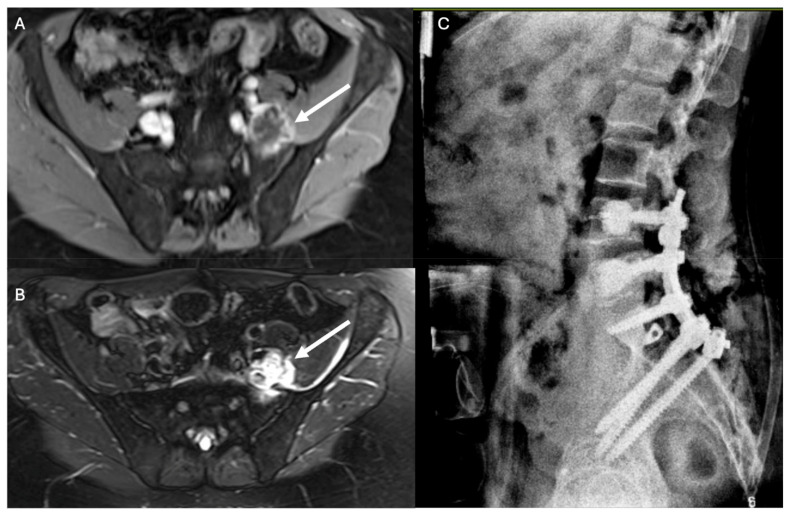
Case 3: pre- versus postoperative imaging. Preoperative T1 post-contrast (A) and T2 (B) MRI showing the left-sided L4 nerve sheath tumor as indicated by white arrow. (C) Postoperative X-ray showing L4–pelvis instrumentation after nerve sheath tumor removal.
